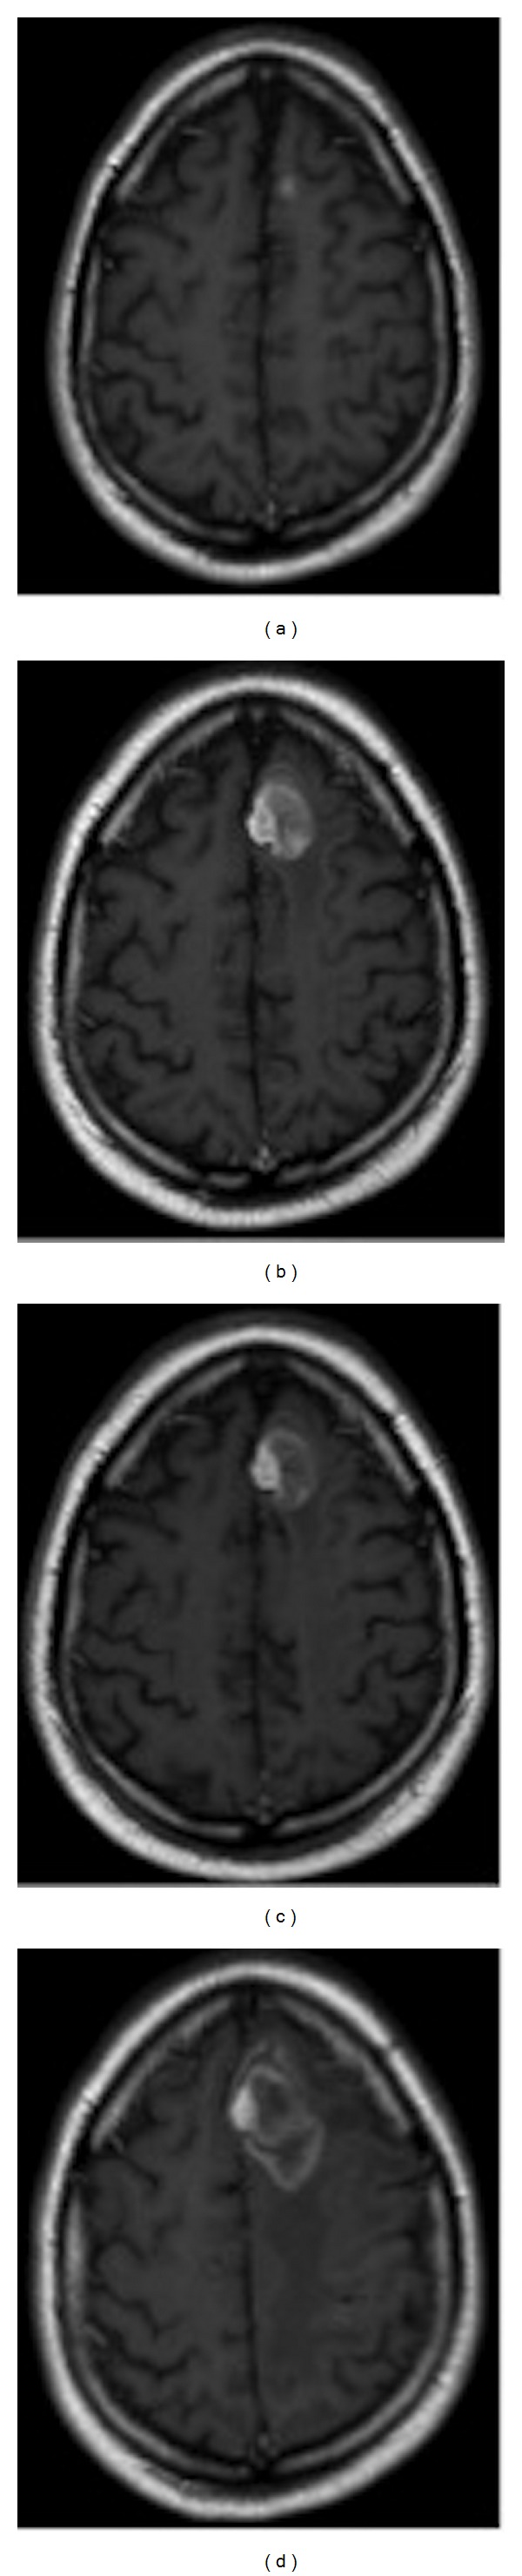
Gadolinium-enhanced T1-weighted MRI images obtained of the MBM of left frontal lobe (a) at diagnosis, (b) prior to SRT, (c) 3 months after SRT, and (d) prior to neurosurgery which revealed RNB only.
